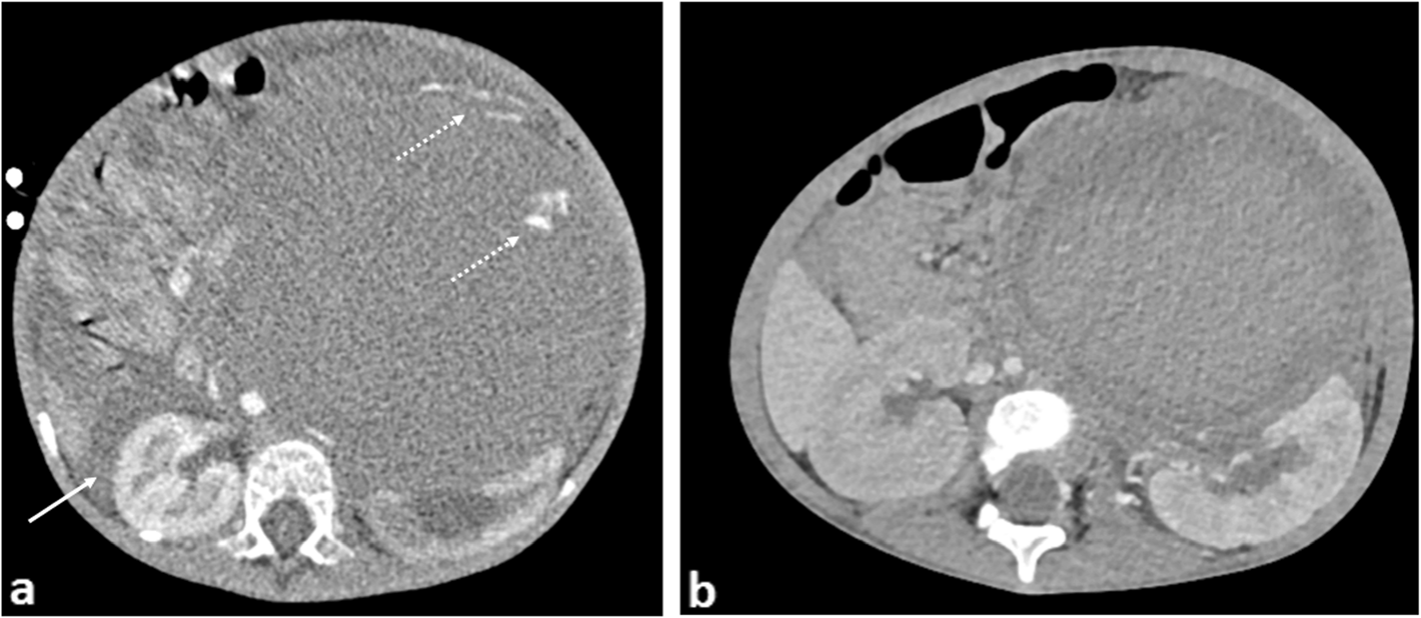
A 3-year-old girl with stage 4 neuroblastoma and acute intra-tumoural haemorrhage. a Axial CT imaging obtained during an episode of acute abdominal pain and drop in haemoglobin shows a large left suprarenal mass with right paracolic free fluid (white arrow) and a blush of contrast within the peripheral left aspect of the mass (dashed arrow) in keeping with active contrast extravasation. b A repeat CT image obtained after vascular embolisation 1 month later reveals reduction in the size of the mass with organisation of the haematoma. The degree of left renal hydronephrosis also appears reduced
